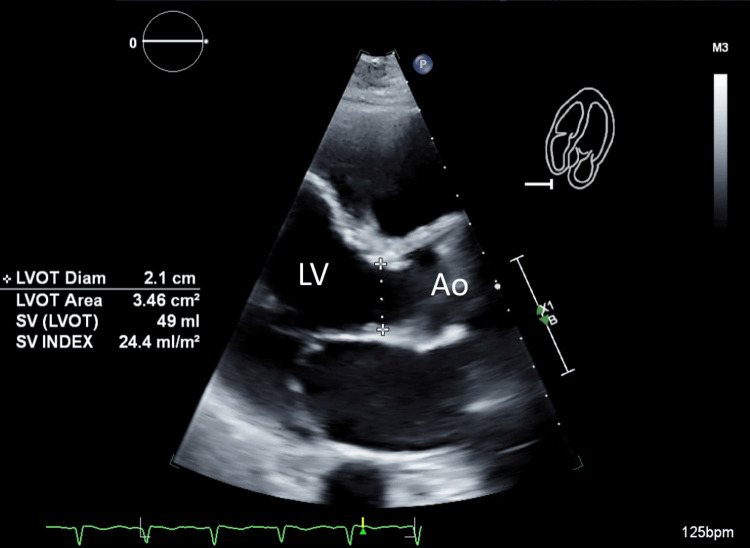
Transthoracic echocardiography (TTE) images showing left ventricular outflow tract Ao: Aorta; LV: Left ventricle; LVOT: Left ventricular outflow tract; SV: Stroke volume.
